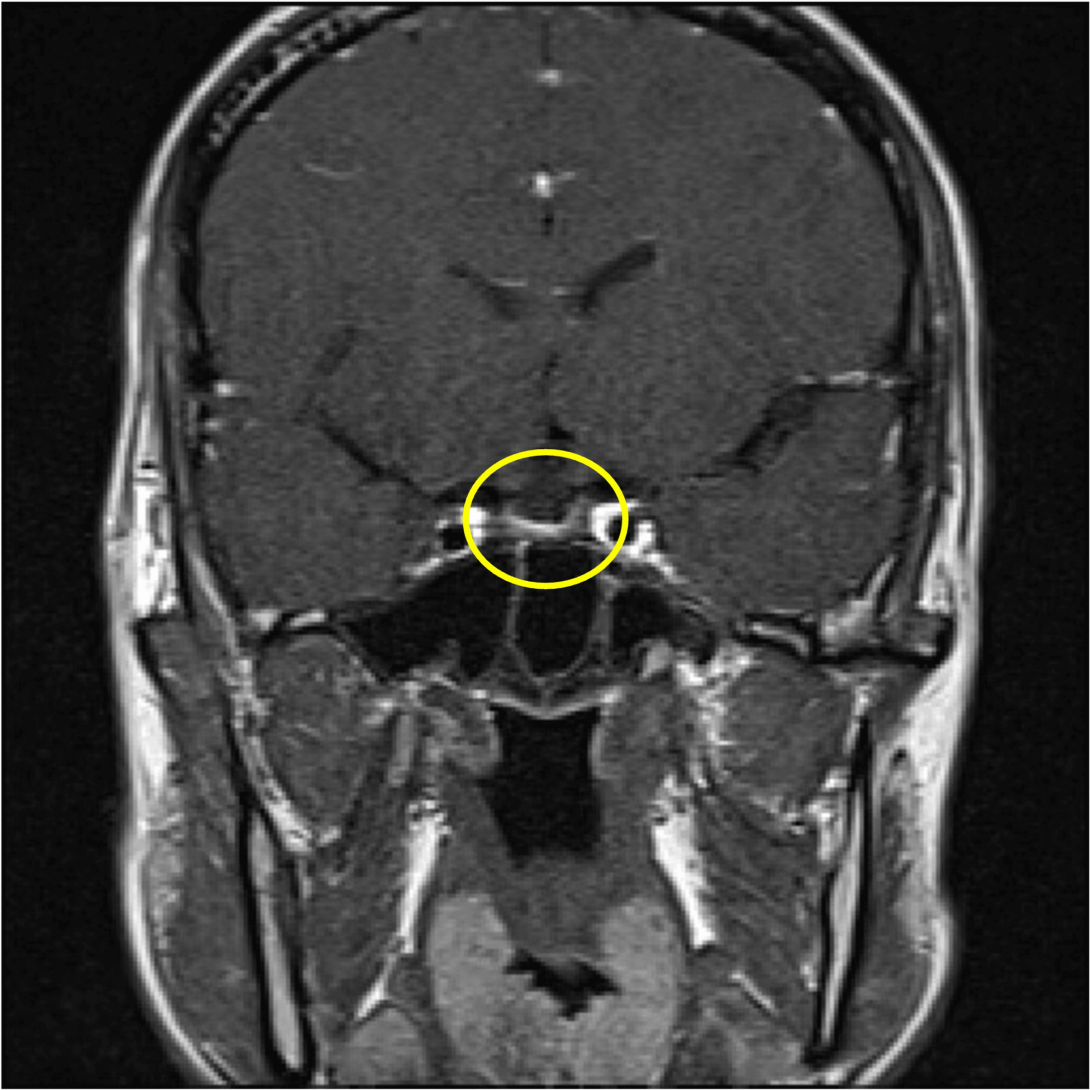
Contrast-enhanced MRI scan of the brain Coronal contrast-enhanced T1-weighted MRI. The pituitary gland shows no signs of enlargement, and no macroadenoma is detected.
